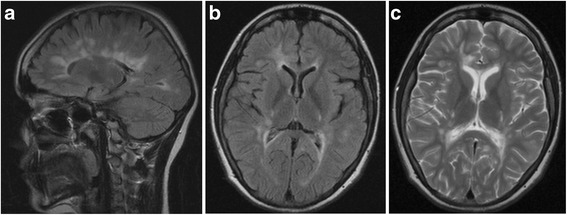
a FLAIR, Fluid-Attenuated Inversion Recovery, sagittal (2006): Periventricular high–signal intensity lesions exhibiting distribution of ovoid demyelinated periventricular lesions radially oriented to ventricles, which is typical for multiple sclerosis. b FLAIR, Fluid-Attenuated Inversion Recovery, transverse (2006): Atypical tumefactive periventricular demyelinated lesion connected to the frontal pole of the lateral ventricle. c T2w, T2-weighted MRI, transeverse (2006): Atypical hyperintensive tumefactive periventricular demyelinated lesion connected to the frontal pole of the lateral ventricle
